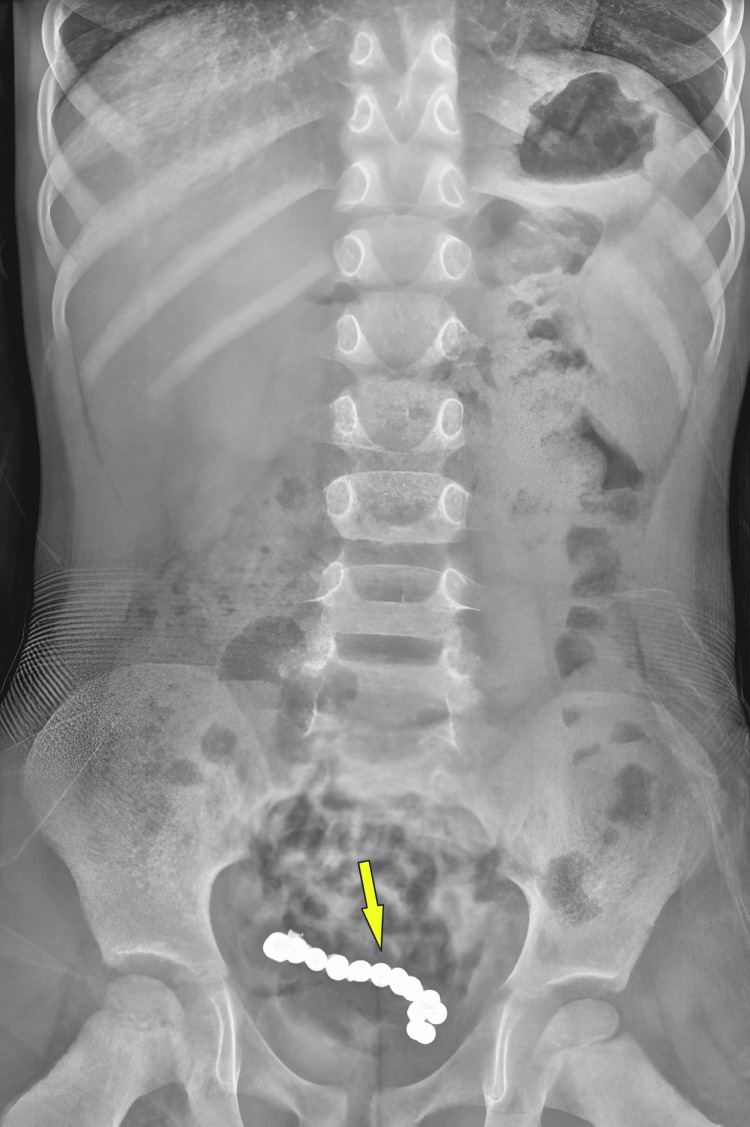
Abdominal radiograph in the erect position showing a well-defined chain of beaded magnets representing a foreign body (arrow)
